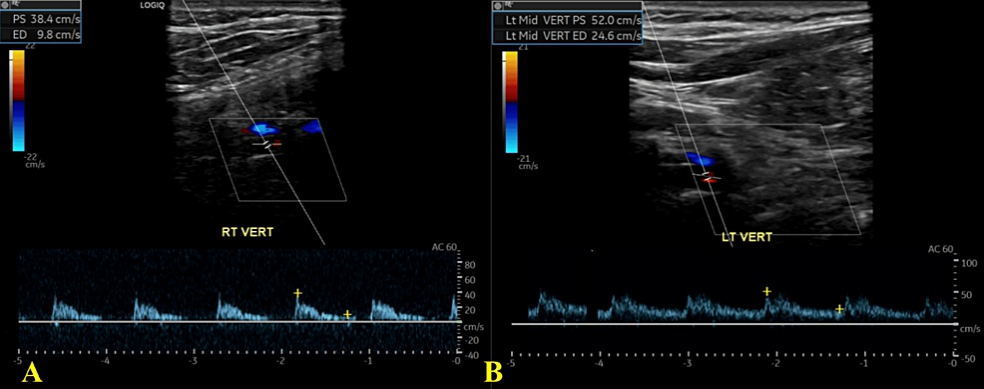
US waveform imaging depicts a high resistance pattern in the right vertebral artery (A) compared to a normal left vertebral artery (B) US: ultrasound
